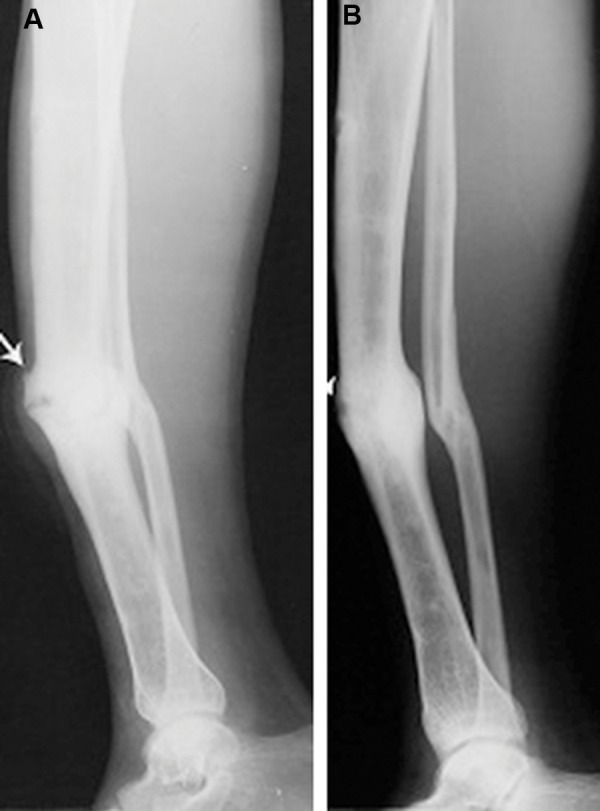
Lateral radiograph of a 32 years male non-united tibial
